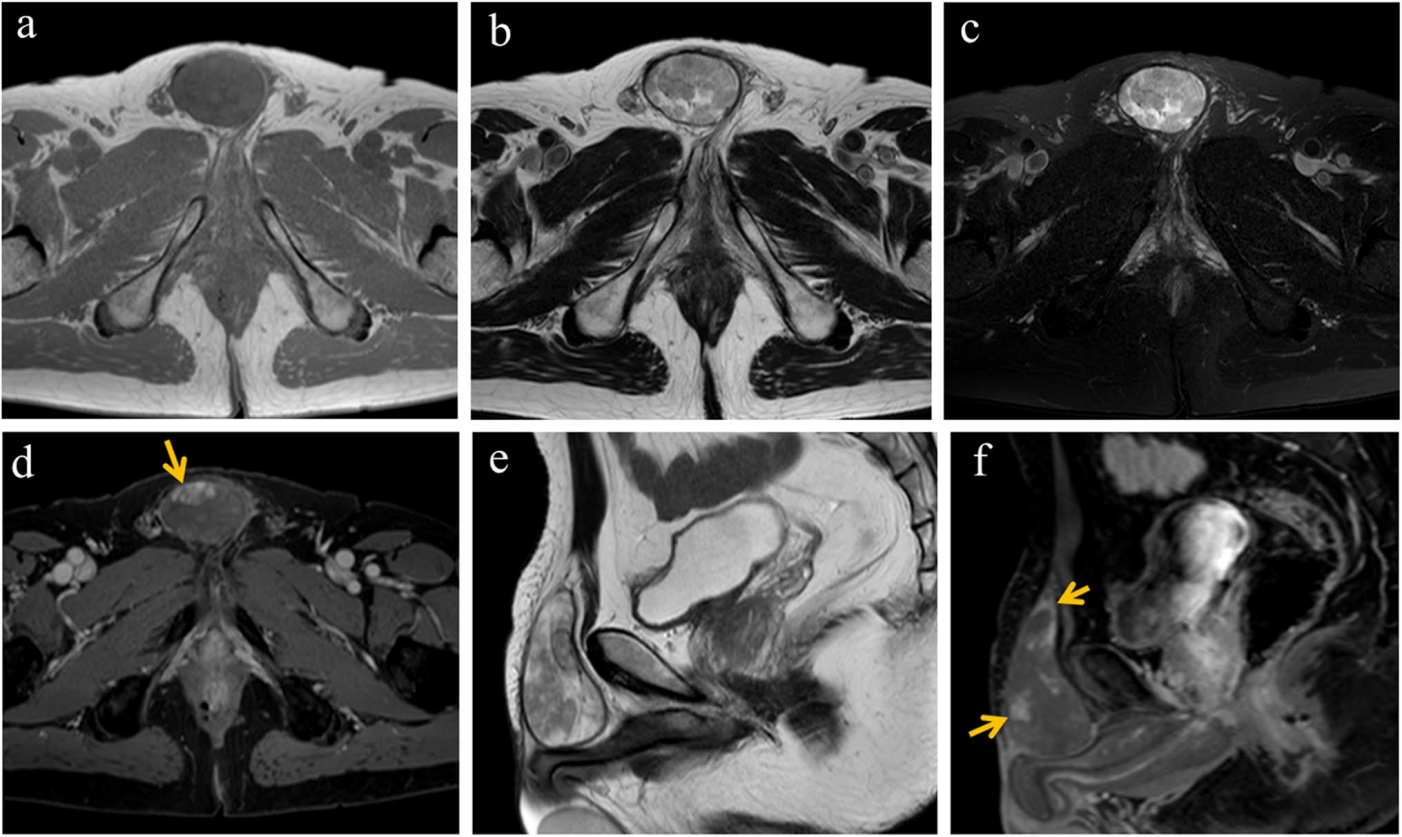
MRI findings of IgG4-related disease. Axial T1WI (a) shows low-intensity signals. T2WI (b, e) and fat-saturated T2WI (c) show an intermediate or hyperintensity with a low-signal capsule. Gadolinium-enhanced fat-saturated T1WI (d, f) shows heterogeneous enhancement (arrows) in the capsule and small areas inside the tumor. MRI, magnetic resonance imaging; T1WI, T1-weighted images; T2WI, T2-weighted images
